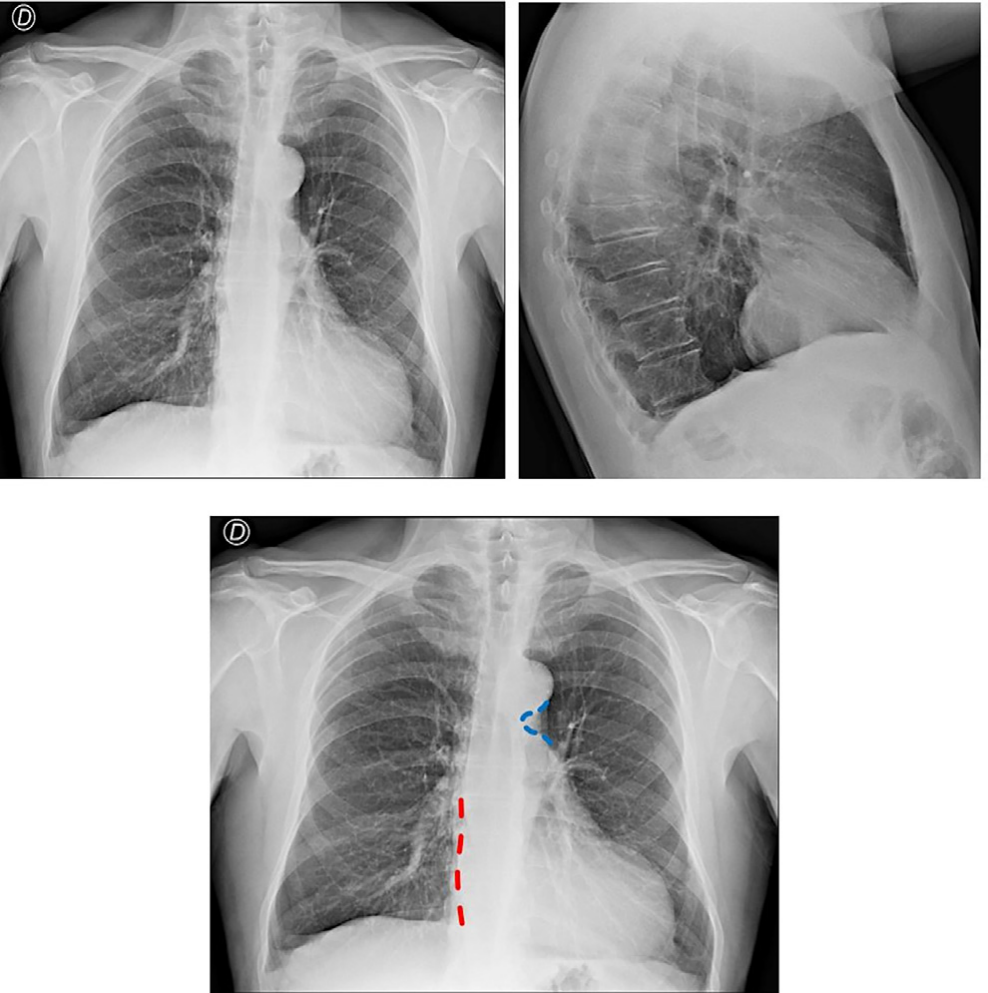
(A) Chest X-ray postero-anterior (PA) and lateral-lateral (LL) projections reported as negative; (B) Chest X-ray *featuring a levopositioned heart with a loss of definition of the rigth second arc border (red dashed line) and a small aortic knuckle with prominent notch (blue dashed line)*.
